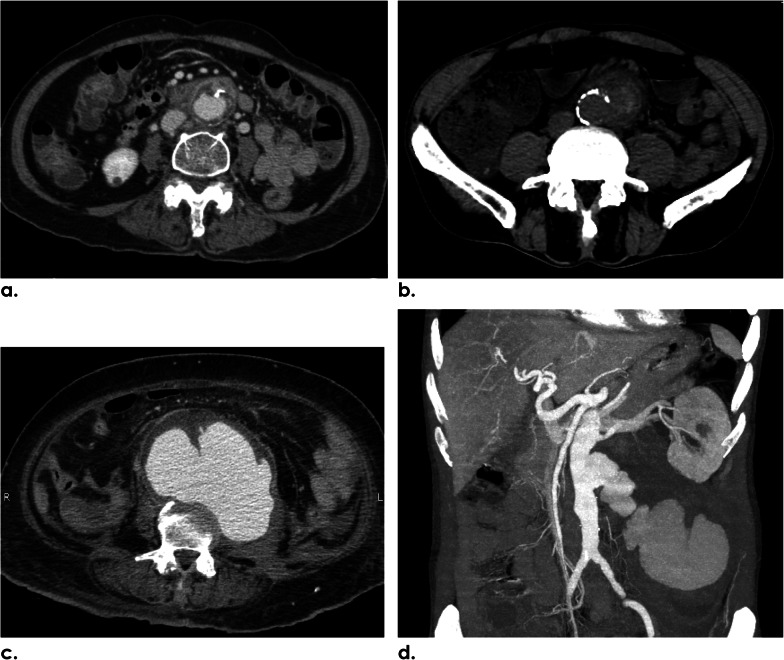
CT findings of INAA instability. a Axial CECT image of a 77-year-old female patient reveals an infrarenal aneurysm and periaortic soft-tissue mass without signs of instability. b Axial NECT image of a 68-year-old male patient depicts hyperattenuation of the peripheral thrombus in an infrarenal aneurysm indicating impending rupture. c Axial CECT of a 61-year-old female patient demonstrates a multilobulated infrarenal aneurysm with its posterior wall draping along the anterior vertebral body, draped aorta sign, representing contained rupture. d Coronal MIP angiographic CT image of a 63-year-old male patient reveals active contrast extravasation from the infrarenal INAA into the retroperitoneal cavity indicating free rupture. *INAA* infective native aortic aneurysm, *CECT* contrast-enhanced computer tomography, *NECT* non-enhanced computed tomography, *MIP* maximum intensity projection
